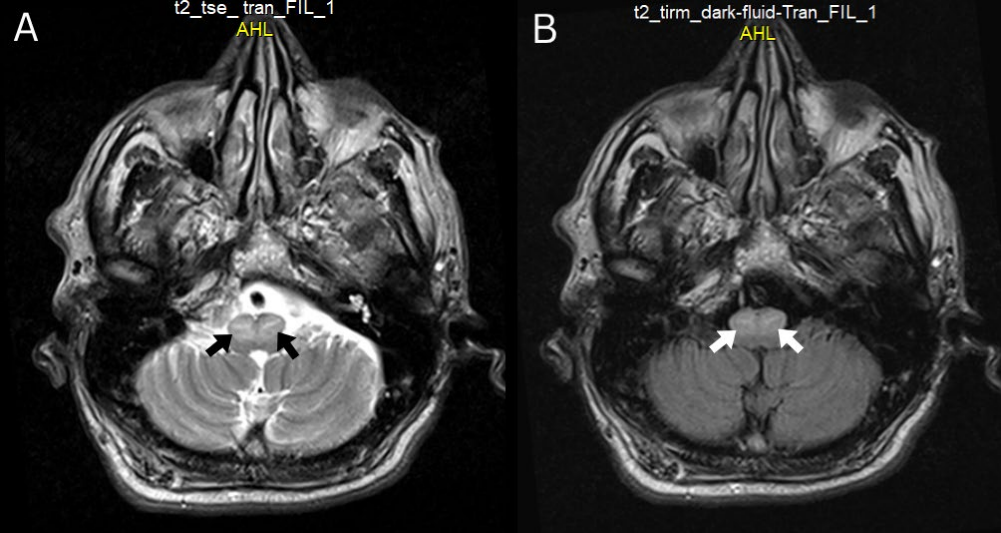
Axial T2‐weighted sequence (A) fluid‐attenuated inversion recovery (FLAIR) sequence (B) showing bilateral hypertrophic olivary degeneration (arrows).
